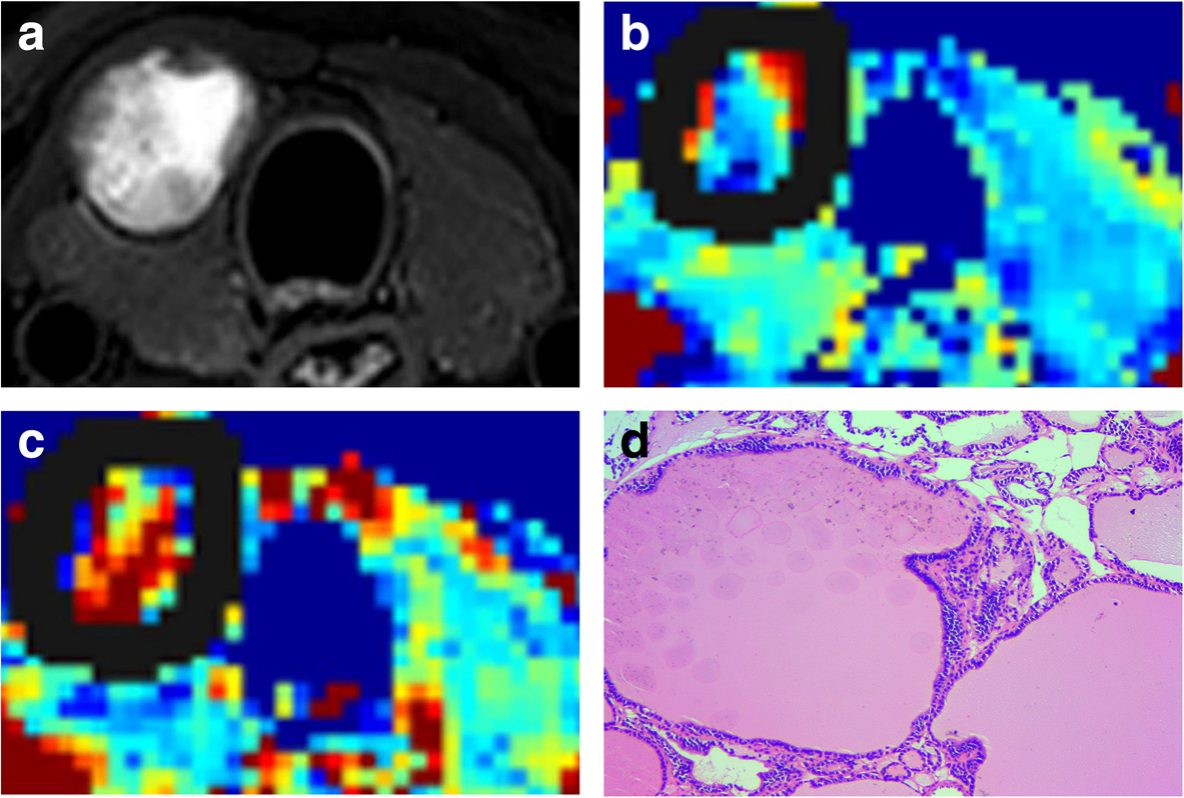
Images of a 36-year-old man with right lobe nodular goitre (central part with cystic necrosis). a. Axial T2-weighted with selected partial inversion recovery (SPIR) image. b-c. IVIM colour map (*D* and *f*). *D* = 1.28 × 10^− 3^ mm^2^/s, *f* = 39.76%. Solid lines show the nodule border containing cystic necrosis. d. Follicles of varying sizes were filled with colloid (HE, × 200)
